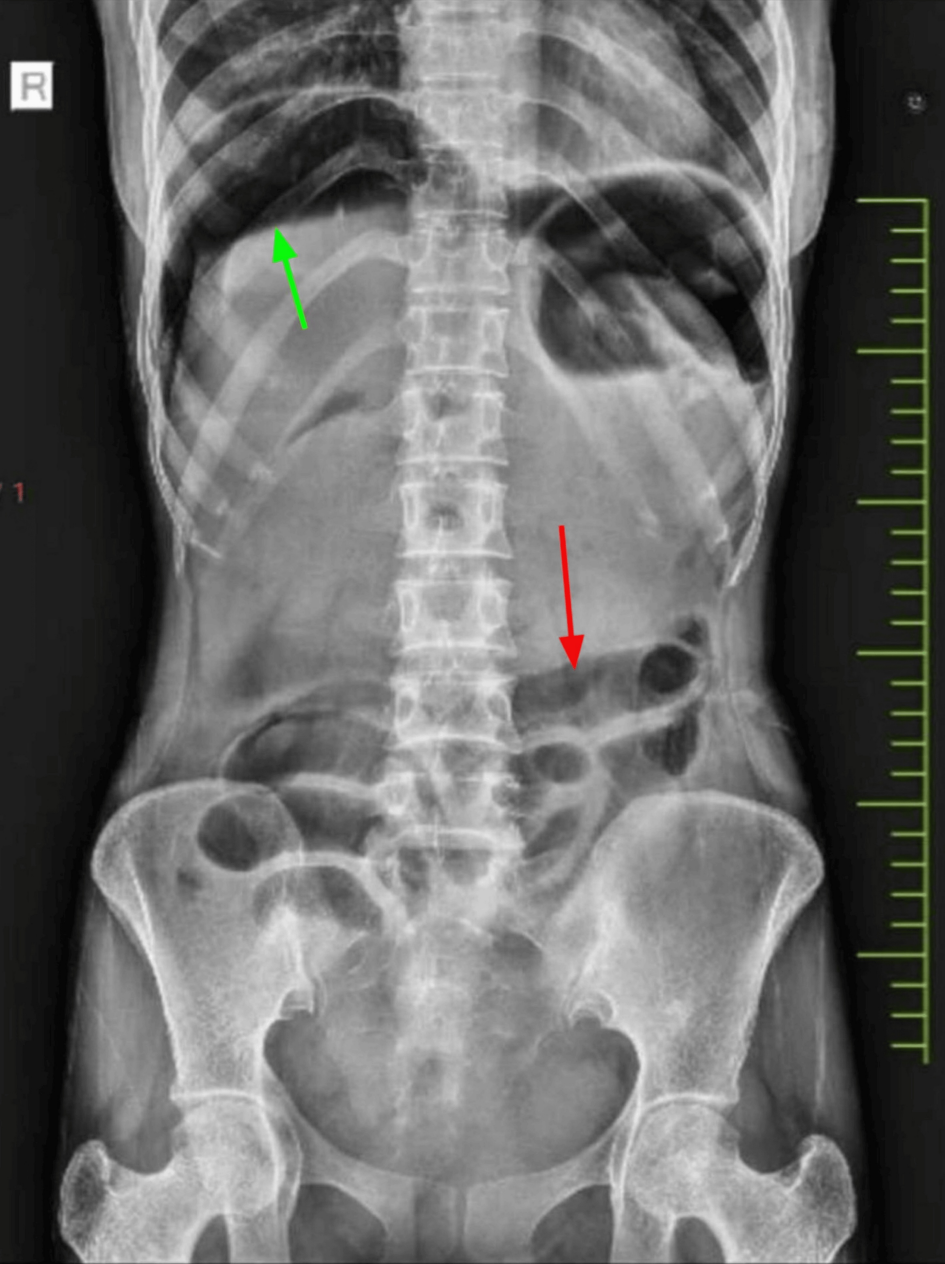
Abdominal radiograph performed 6 hours after colonoscopy revealed free gas under the right dome of the diaphragm (green arrow) with distended bowel loops (red arrow).
